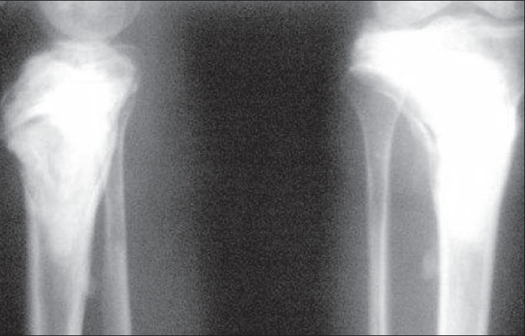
X-ray (lateral and AP view) after 1 year shows no evidence of recurrence
